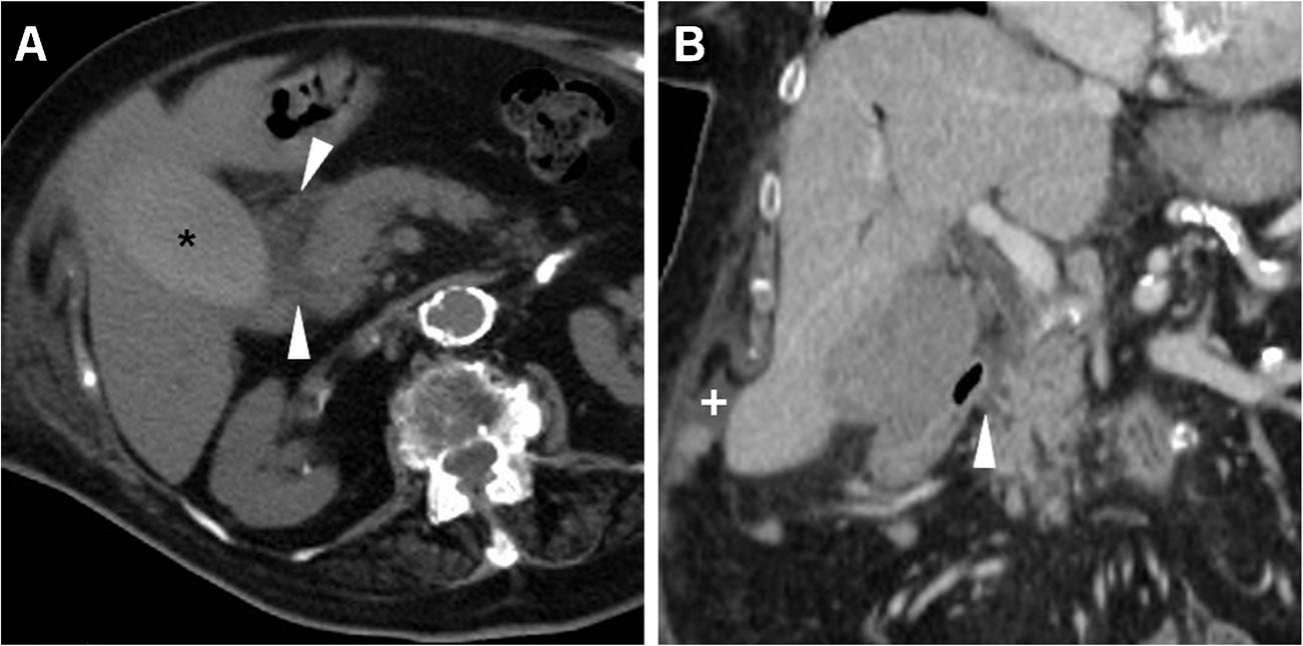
Oedematous acute pancreatitis (*AP*) in a 77-year-old female 3 days after endoscopic treatment of choledocholithiasis including sphincterotomy. Unenhanced (a) and post-contrast (b) CT images showed persistent moderately hyperattenuating contrast medium (*) in the gallbladder, oedema and fluid in the pancreatico-duodenal groove (*arrows*), minimal ascites (+) consistent with clinical and laboratory features of mild AP, and excluded abnormal collections and non-enhancing parenchyma indicating pancreatic necrosis. The patient had an uneventful course with conservative treatment
